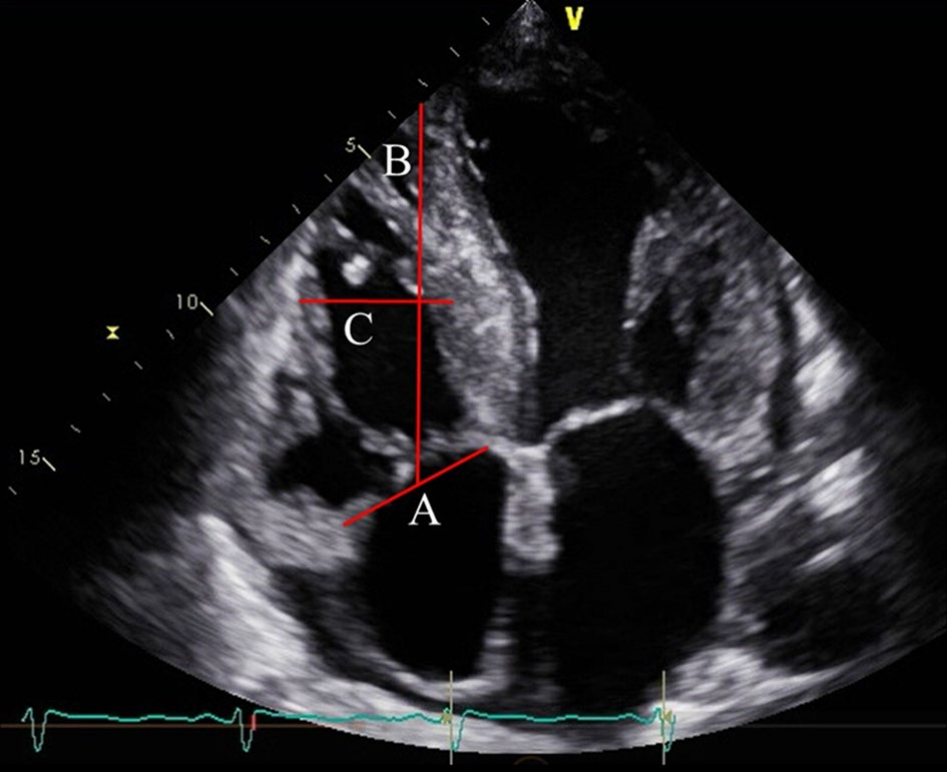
Definition of RV sphericity index. This echocardiographic image in apical four chamber view indicates measurement of RV sphericity index and tricuspid annular diameter. Initially, the tricuspid annulus diameter during the end-diastolic phase was measured (line A). Subsequently, a line was drawn connecting the RV apex to the midpoint of line A (line B). Finally, a perpendicular line was depicted connecting the RV free wall and septum, intersecting the midpoint of line B (line C). The RV sphericity index was calculated as the ratio between line C / line B. RV, right ventricular.
